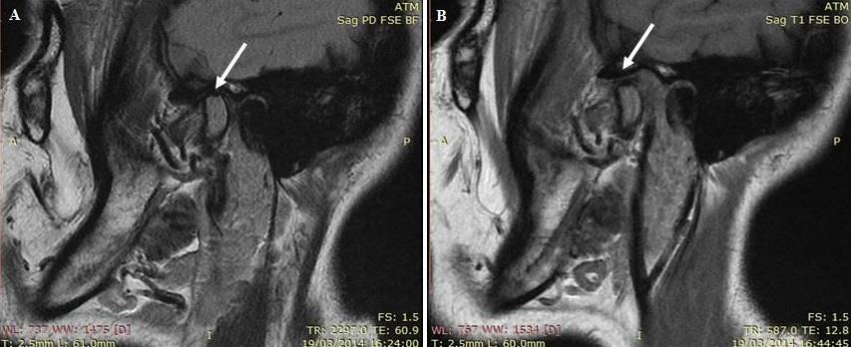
Magnetic resonance imaging (MRI) sagittal slices: A) MRI in closed-mouth position showing mandibular condylar process resorption, joint space decreased anddegenerative alterations of the disk; B) MRI in open-mouth position showing hypomobility of mandibular condylar
